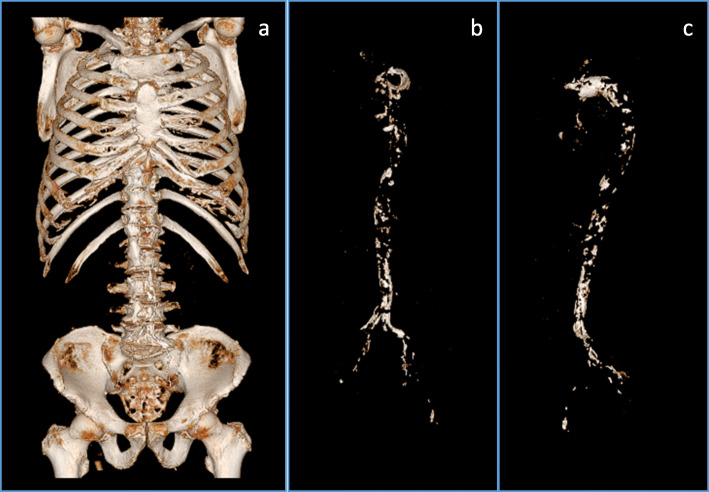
Representative image of vascular calcification using 3D imaging software. 3D images from an original CT image were reconstructed (a). After the removal of bone and the manual confirmation, the vascular calcification image is shown in (b). Rotation of the vascular calcification image (b) is shown in (c)
